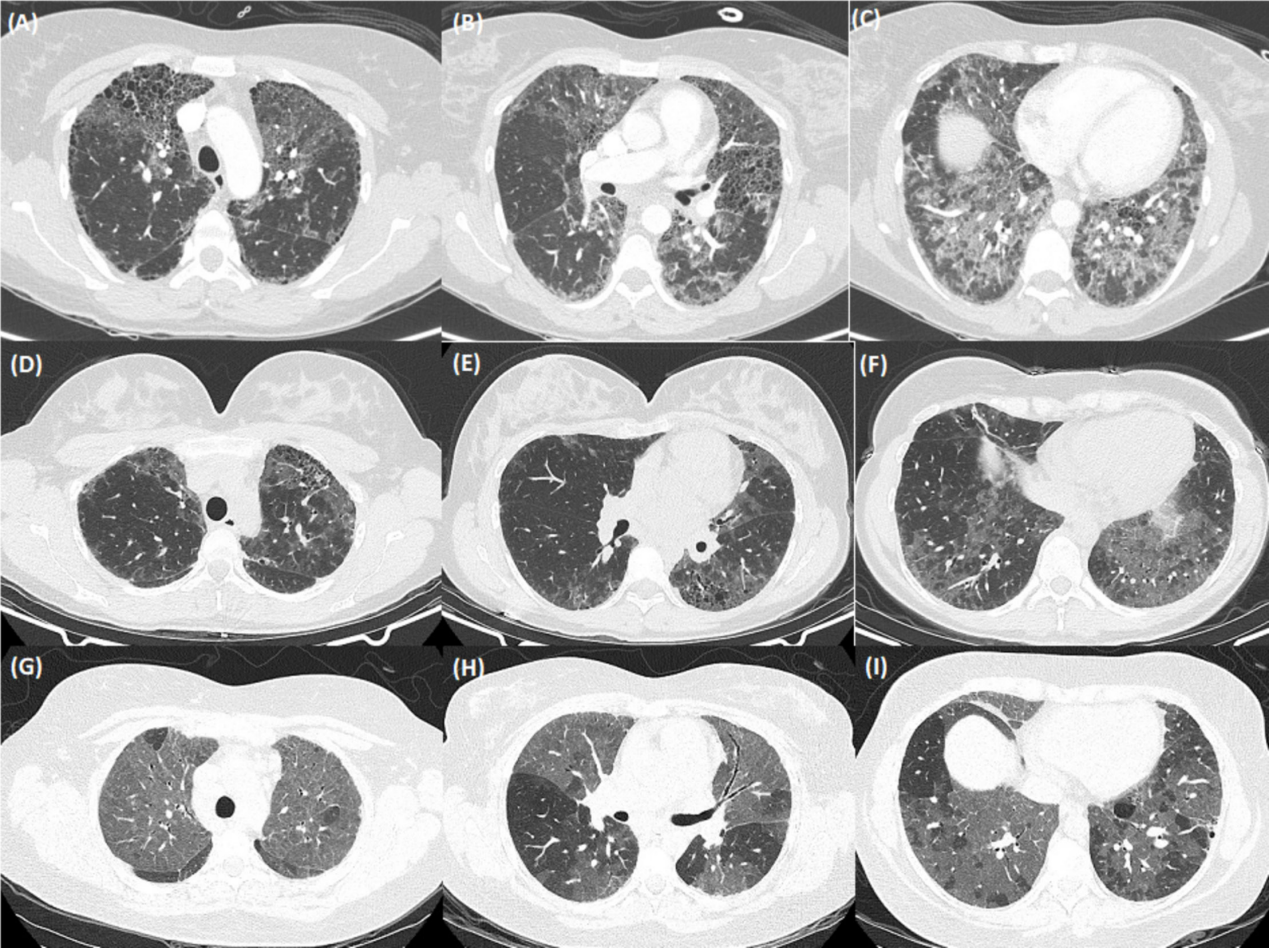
Case series of three females with ILD secondary to ABCA3 variants. Representative CT slices at presentation from (A–C) Case 1, (D–F) Case 2, and (G–I) Case 3.
